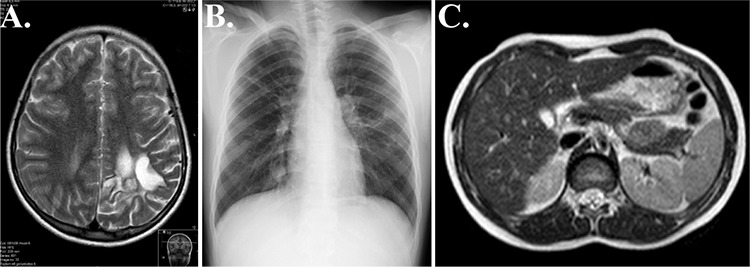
Repeat images after 56 months of albendazole therapy: Encephalomalacic changes were observed at the site of surgery on postoperative T2-weighted axial image (a). Chest radiography showing complete regression of hydatid cysts (b). T2-weighted axial image of the liver with total regression of hydatid cysts (c).
